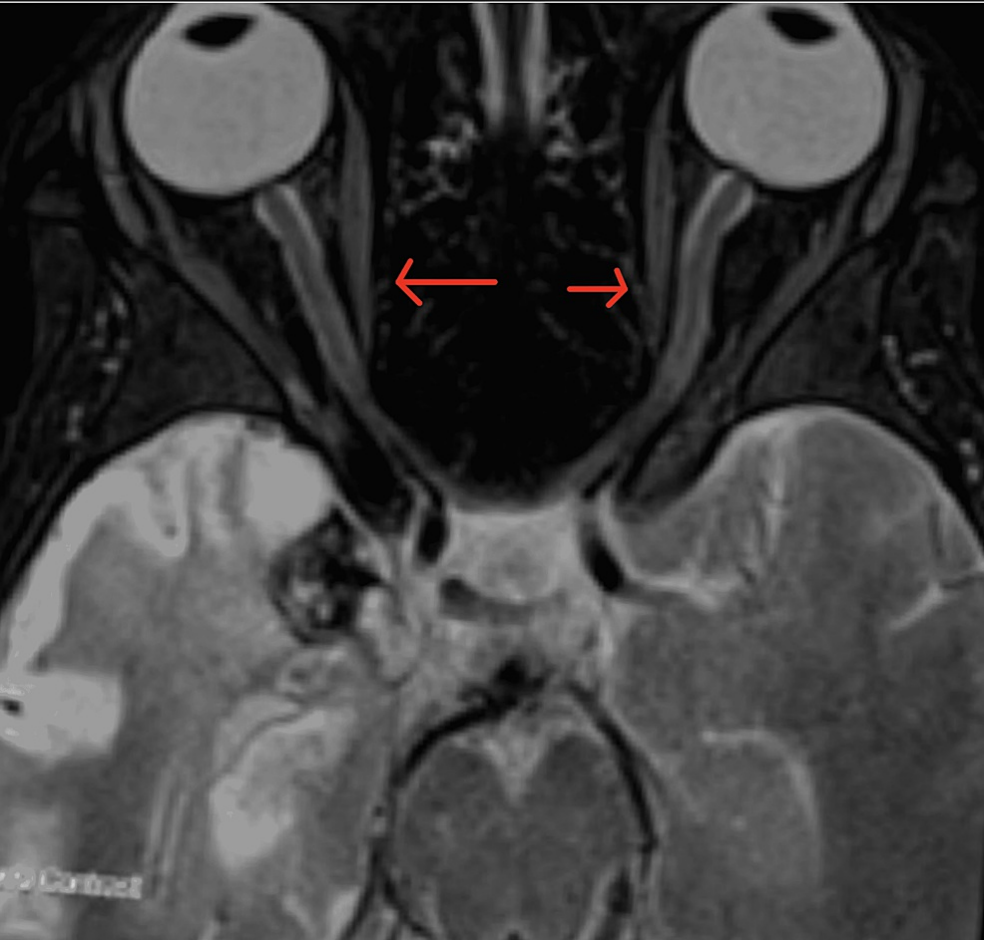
MRI brain without contrast focused on orbits, T1-weighted, axial view The axial view shows bilateral ocular globes and extra-ocular muscles that are normal.
